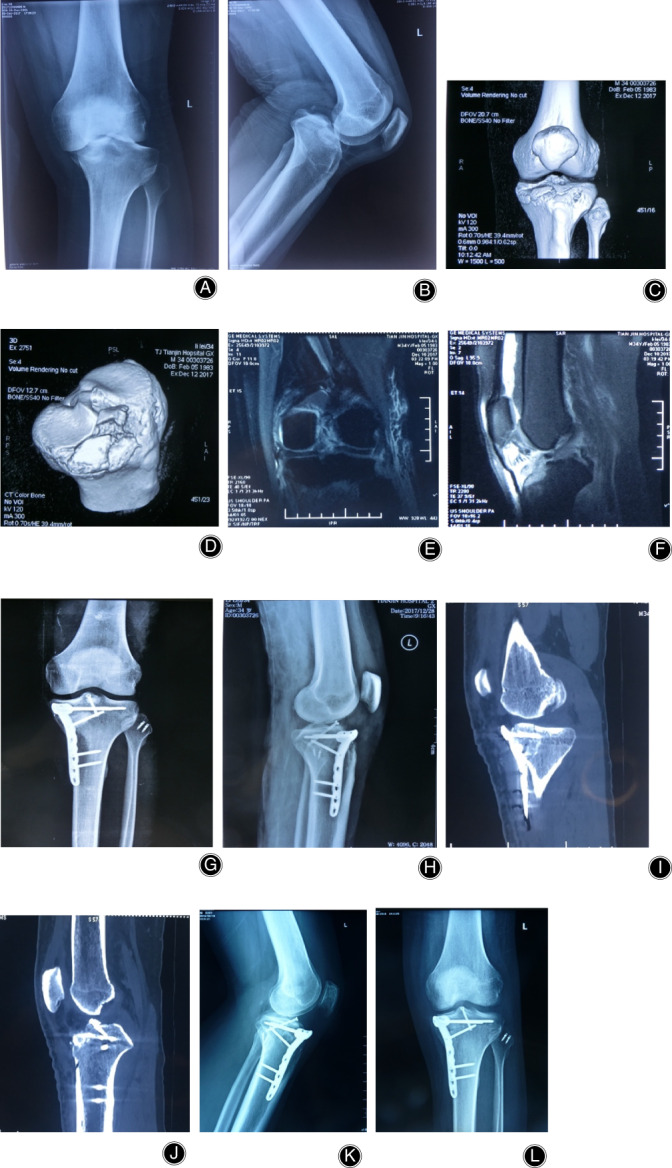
Anteromedial tibial plateau fracture. Male, 34 years old. (A, B) X‐Ray shows that the medial tibial plateau had a displaced fragment and location of the knee. (C, D) 3D CT showed the anteromedial tibial plateau fracture, compression of the articular surface, and avulsion fracture of tibial intercondylar eminence. (E, F) MRI showed the lateral collateral ligament (LCL) injury and avulsion fracture of the ACL. (G, H) The anteromedial articular surface were reduced and fixed with “T” locking plates. The fracture of the tibial intercondylar eminence was fixed with screws. The LCL injury was repaired with anchoring sutures. (I, J), Postoperative CT showed anatomical reduction of the fracture. (K, L) The fracture healed with anteromedial reduction 1 year after the operation.
